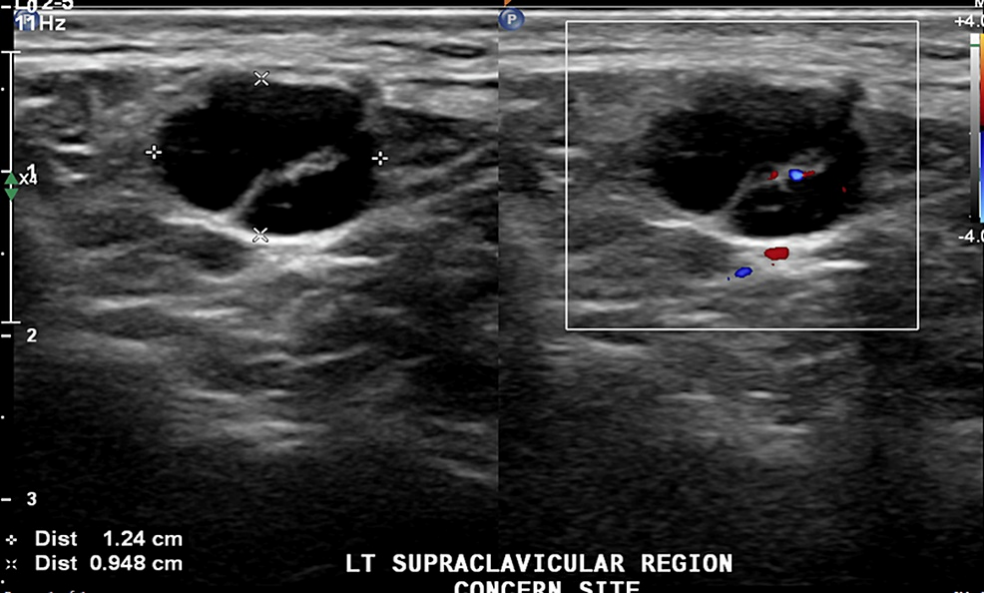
Grayscale (left) and color Doppler (right) ultrasound of the left supraclavicular region shows enlarged round lymph nodes with preserved fatty hilum and normal vascularity, measuring 9.5 mm in the short axis.
